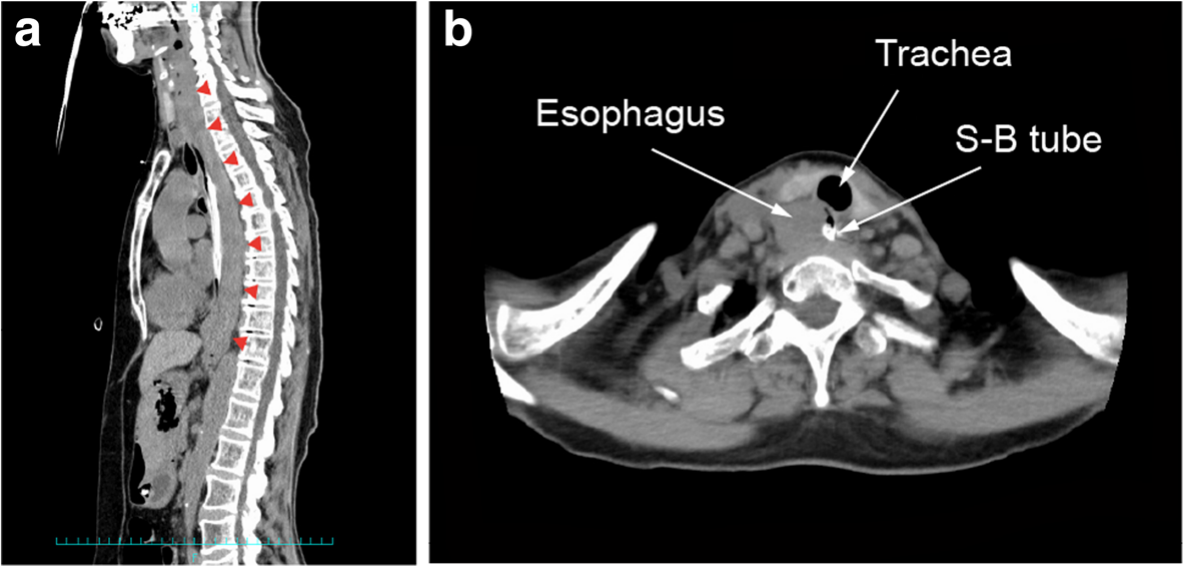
Cervical and thoracic CT images after insertion of Sengstaken-Blakemore tube. a Sagittal section: the soft tissue shadow is filled and dilatation can be seen in the posterior wall over the entire length of the esophagus (red arrows), ventrally displacing the lumen. b Cross-section: the cervical esophagus is dilated, displacing the trachea toward the left side
